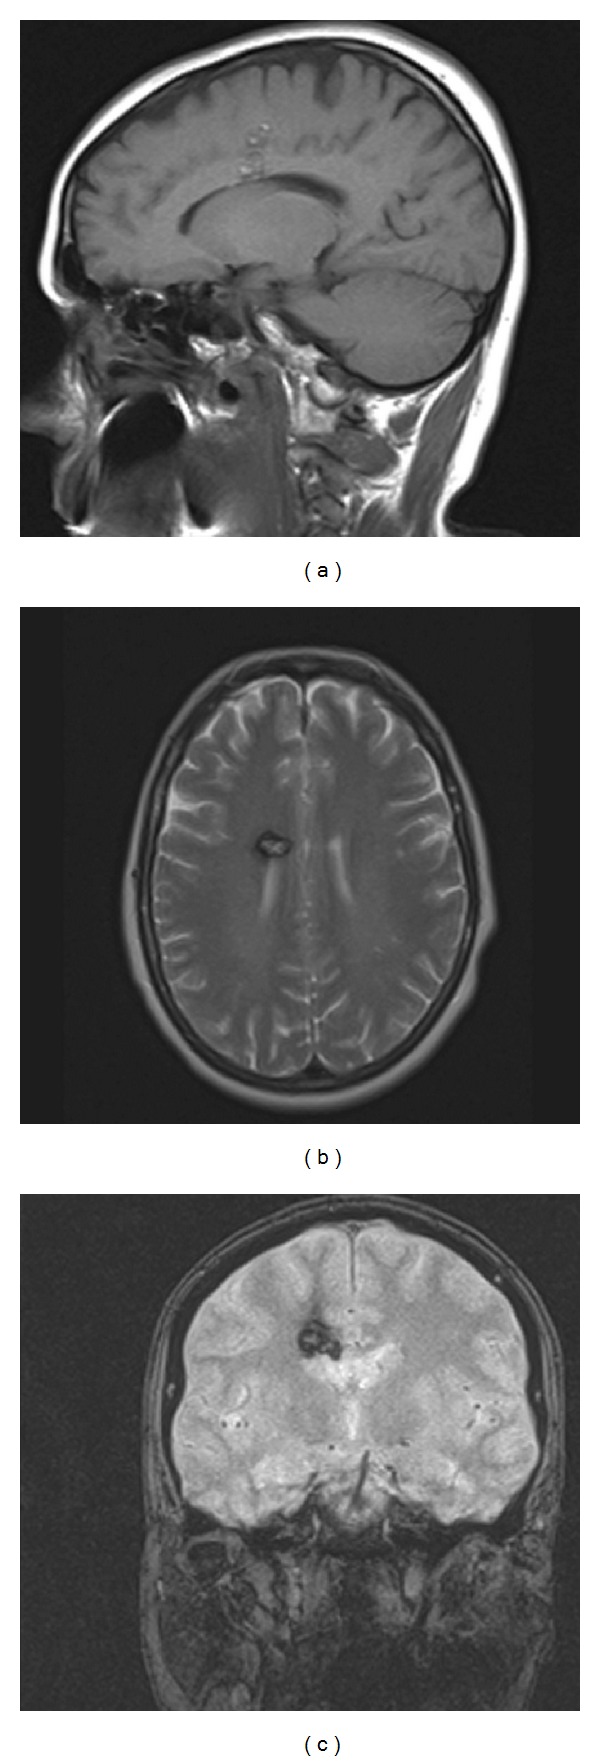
(a) Sagittal T1-weighted MR image showing the cavernous angioma embedded in the middle-third of the corpus callosum and extending into the cingular cortex. Axial T2-weighted (b) and coronal gradient echo (c) MR images evidence signs of a recent intralesional bleeding of the right-sided cavernous angioma.
